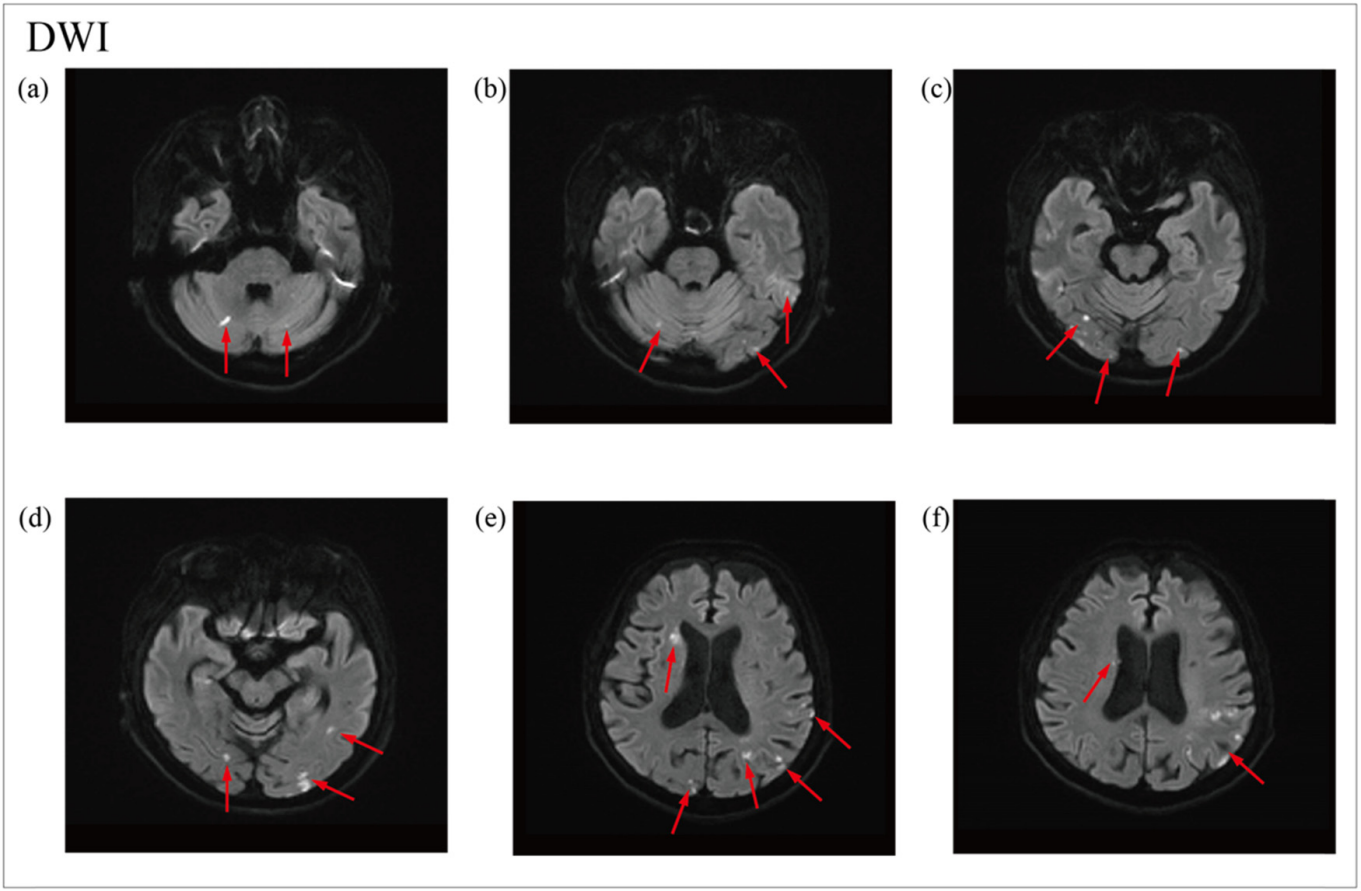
DWI sequence of cerebral MRI. (a–f) Multiple lesions of acute lacunar cerebral infarcion in cerebral cortex, subcortex and cerebellum.
